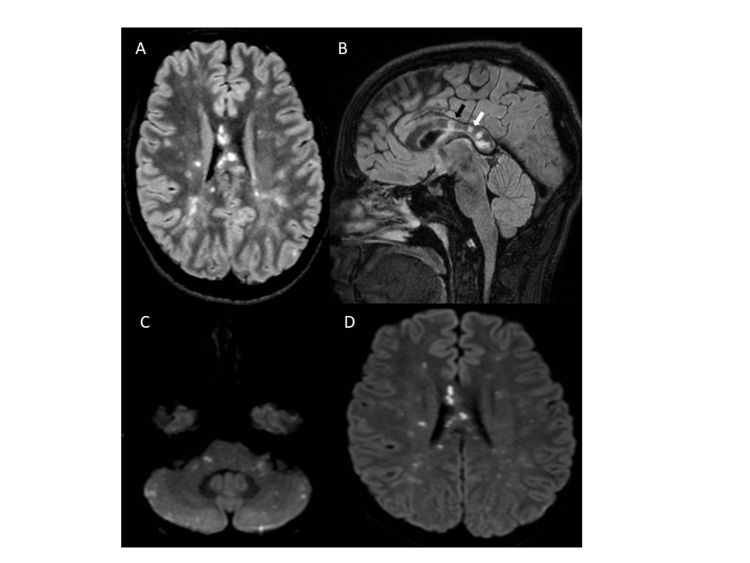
Cerebral MRI images on admission (A-D) (A) Periventricular, subcortical and callosal lesions in the axial FLAIR section. (B) Characteristic snowball (white arrow) and “icicle” (black arrow) lesions in the corpus callosum. C and D. Supratentorial and infratentorial small acute ischemic lesions on DWI sequence.
